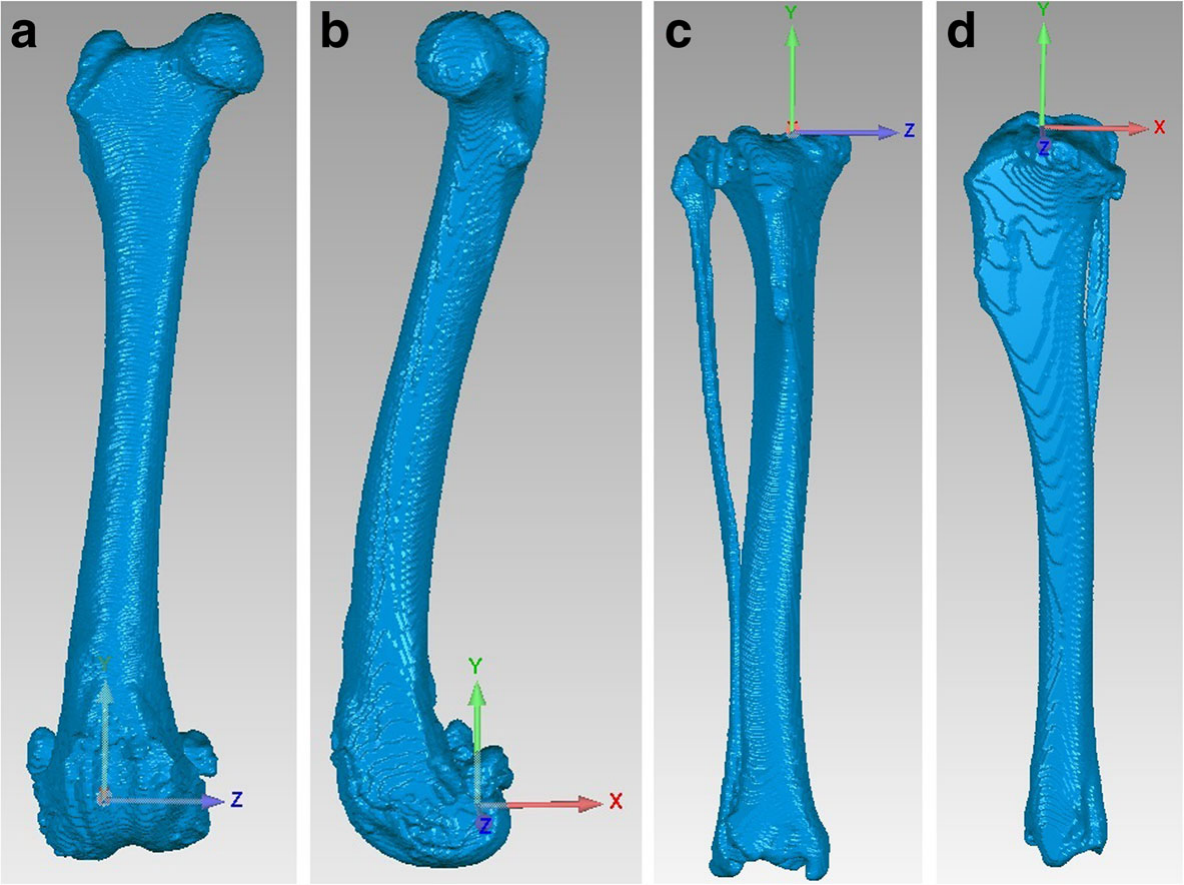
Femoral and tibial coordinate systems. CT-generated 3-dimensional digital models of the femur (craniocaudal (1a) and lateral (1b) views) and tibia (craniocaudal (1c) and lateral (1d) views) with 3-dimensional coordinate system applied
